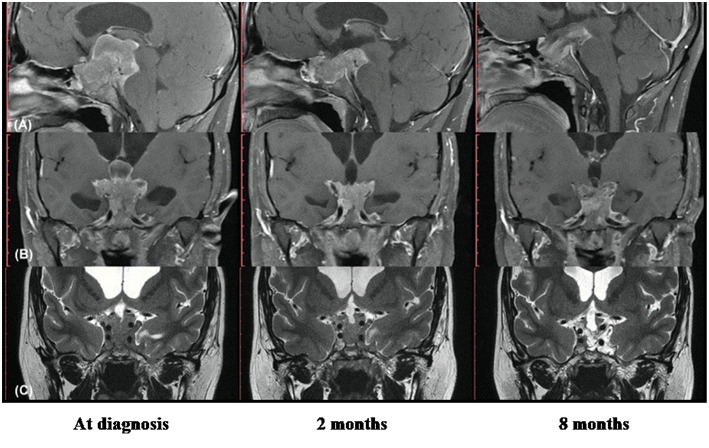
Radiological images showing remarkable therapeutic response with cabergoline in young man with hydrocephalus and intracranial hypertension for giant prolactinoma as first clinical manifestation of multiple endocrine neoplasia type 1. (A) Magnetic resonance imaging (MRI) in T1 with gadolinium, sagittal section; (B) MRI in T1 with gadolinium, coronal section; (C) MRI in T2 with gadolinium, coronal section. Left images show the solid-cystic sellar and parasellar tumoral mass identified at diagnosis (7.5 × 4.4 × 3.6 cm), with invasion of cavernous sinus, midbrain and third ventricle, occluded foramen of Monro and moderate to severe supratentorial obstructive hydrocephalus; Central images obtained 2 months after the beginning of cabergoline shows significant reduction of the tumor. Right images, with 8 months of cabergoline reveal a notable tumoral shrinkage and necrosis of the tumor.
